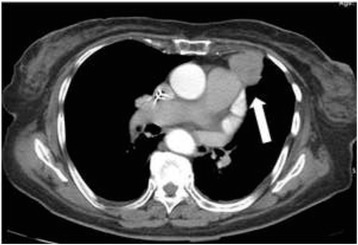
Preoperative CT scan of the chest. Incidentally discovered 5.8 cm anterior mediastinal mass noted on preoperative CT scan. The arrow points to the tumor
